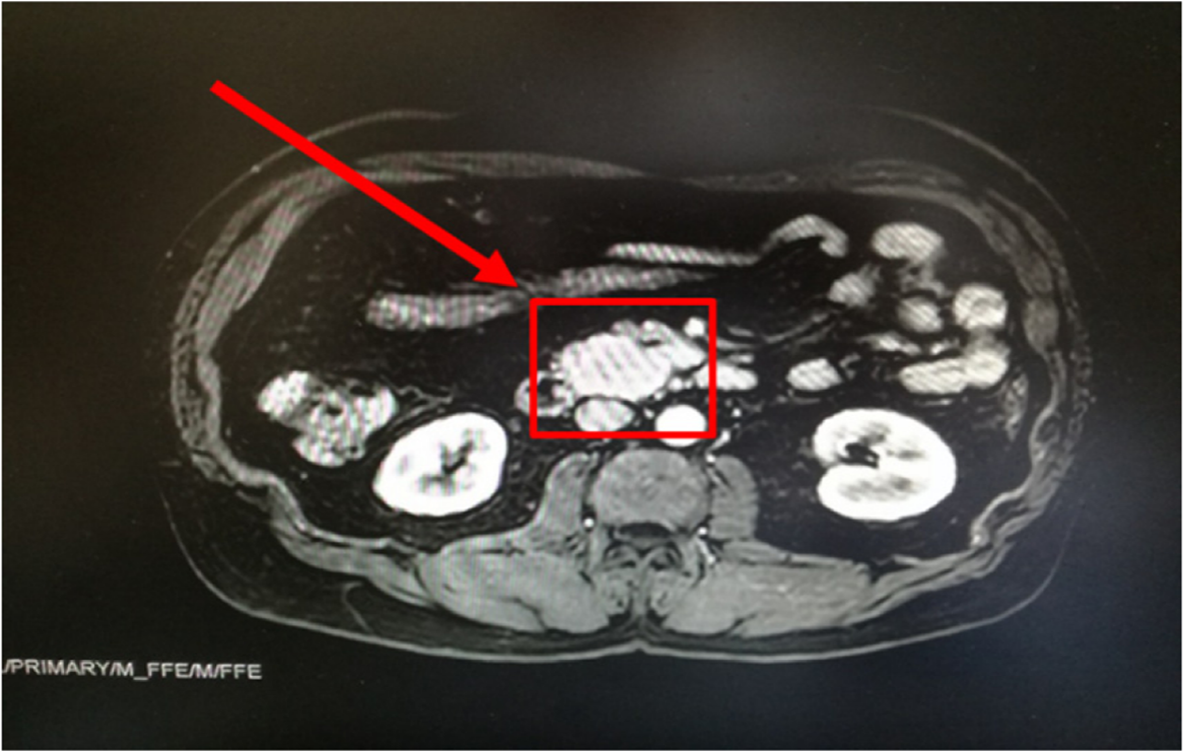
Abdominal MRI showing a solid tumor with definite margins of the uncinate pancreatic process of 4x3x3.5 cm
